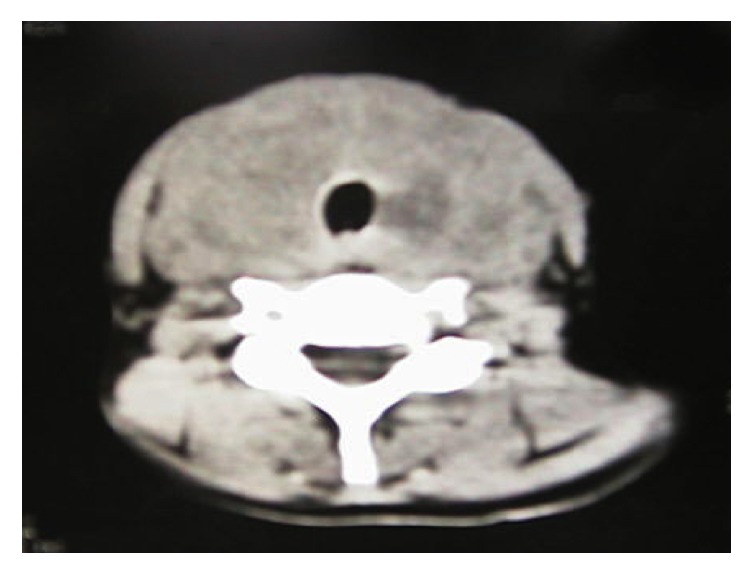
Axial computed tomography showing diffuse infiltrate with no precise boundaries around the thyroid.
